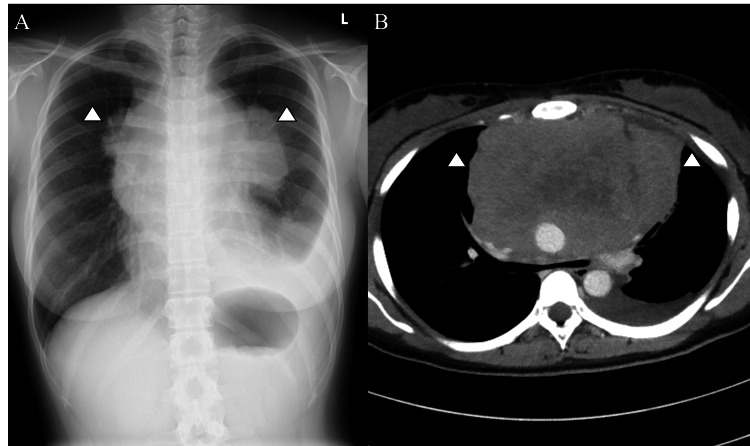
Imaging findings on the day of admission A. Chest radiograph on the day of admission. B. Contrast-enhanced CT on the day of admission The images showed a massive anterior mediastinal tumor with internal necrosis compressing the carina, bilateral main bronchi, and pulmonary arteries CT: computed tomography
